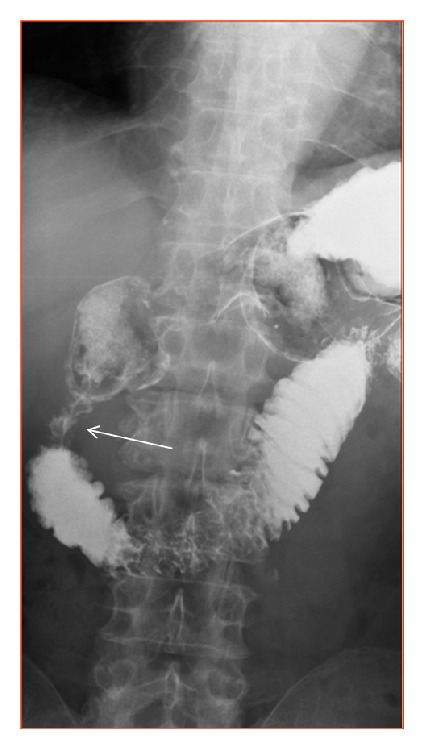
Stomach and duodenum X-ray with barium contrast. Patient 51 y.o. Duodenal dystrophy. Stenosed vertical branch of the duodenum (arrow).
